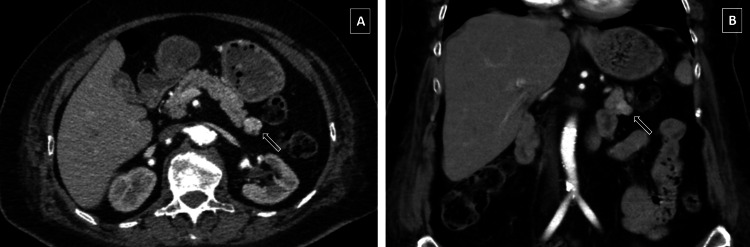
Contrast-enhanced CT scan of the abdomen. The white arrow points to the nodular lesion located in the tail of the pancreas. (A) Axial view. (B) Coronal view.
